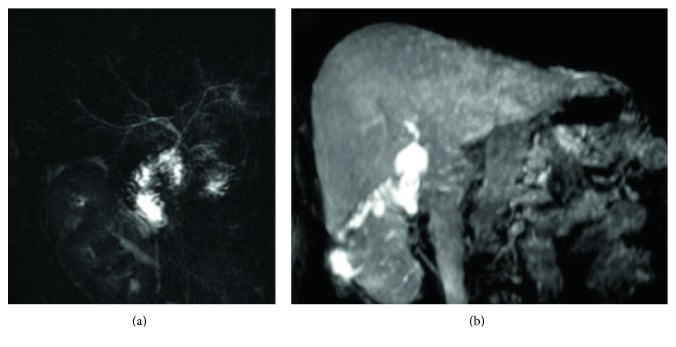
Patient with a biliary-enteric anastomosis, performed after duodenopancreatectomy. 2D T2-weighted cholangiography (a) shows normal appearance of a biliojejunal anastomosis. Excretory phase—obtained after contrast administration of Gd-BOPTA—confirms regular opacification at the surgical connection (b).
